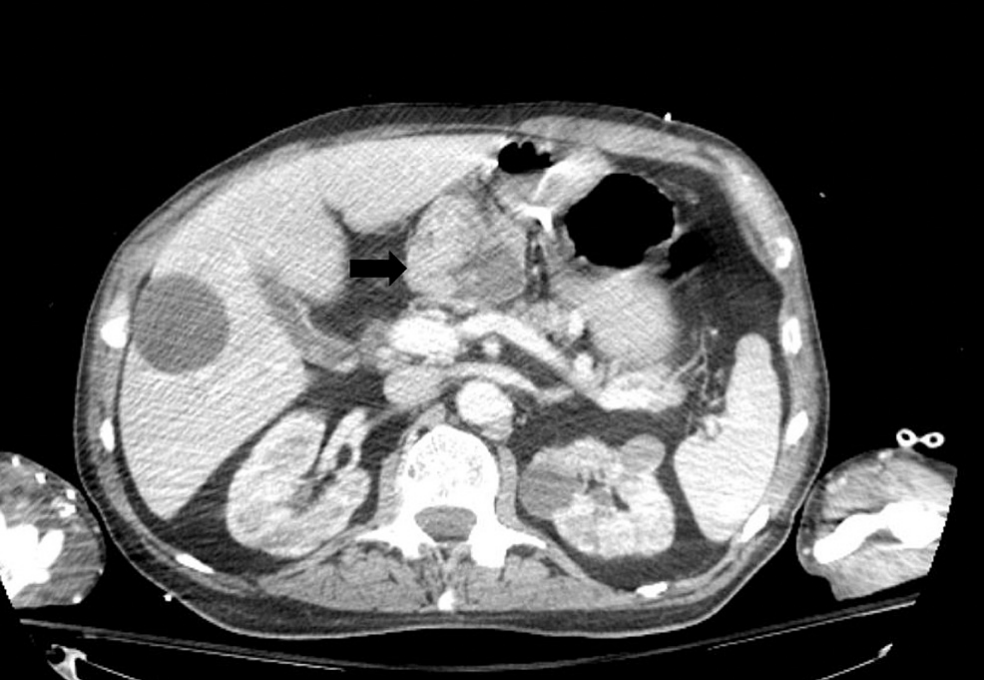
Exophytic solid and cystic gastric mass. Axial CT with contrast revealed a mixed solid and cystic 6.2 cm x 6.1 cm exophytic gastric mass (black arrow) that was located distal to the stomach. This mass was previously determined to be a GIST by biopsy. CT also revealed low-attenuation lesions in the liver and spleen, which were most likely cysts, and multiple small renal cysts along with heterogenous attenuation with a nodular surface, which was most likely secondary to chronic scarring. GIST, gastrointestinal stromal tumor
